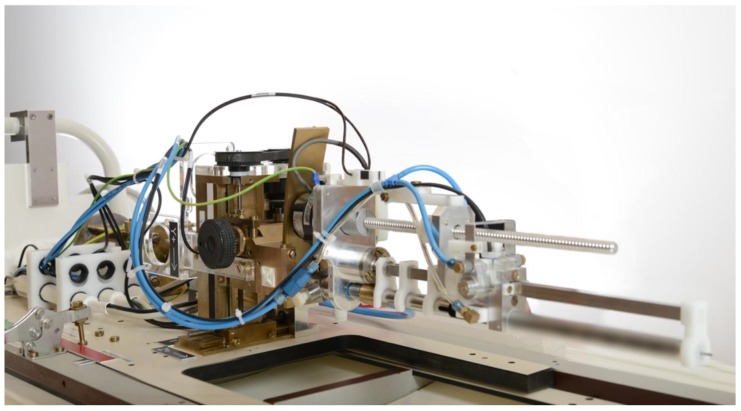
Magnetic resonance imaging (MRI)-compatible robotic implantation device for prostate brachytherapy. A cylindrical weight that is pneumatically driven hits the needle holder to tap a brachytherapy needle into the prostate. When placed between the patient’s legs inside an MRI scanner, the needle can be tracked using live images.
